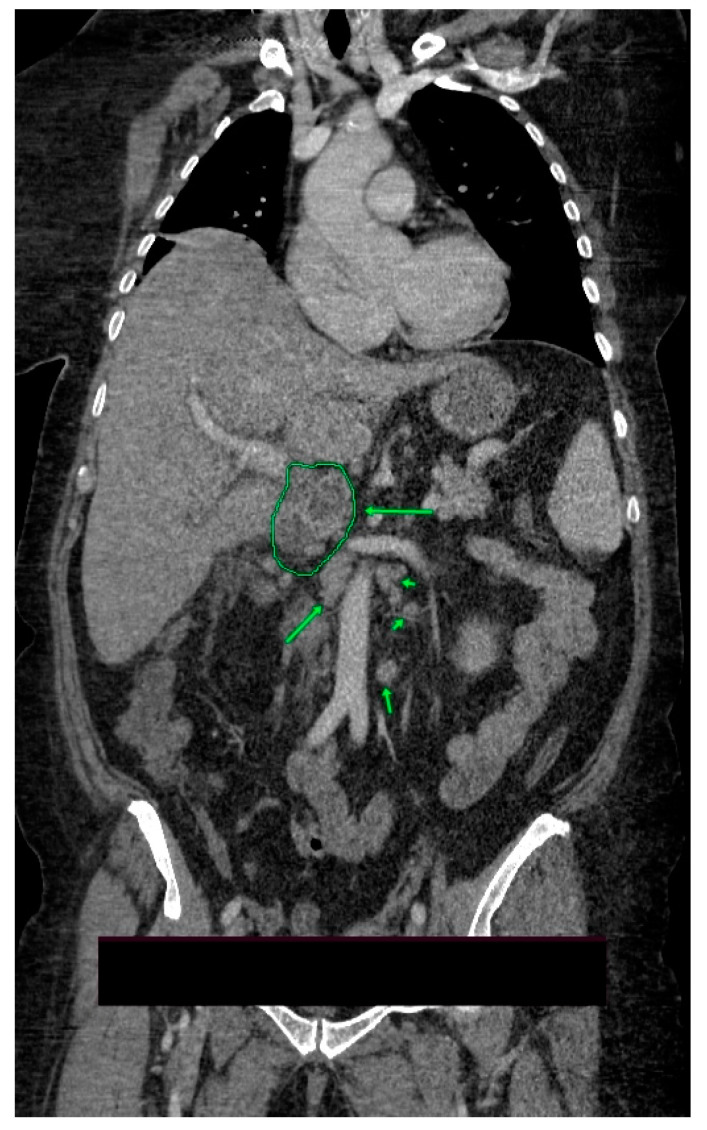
Contrast-enhanced abdominal pelvic computed tomography, venous phase, coronal imaging plane: globally enlarged, irregularly shaped, inhomogeneous cephalic contours of the pancreas, multiple periaortic and interaortocaval lymph nodes.
